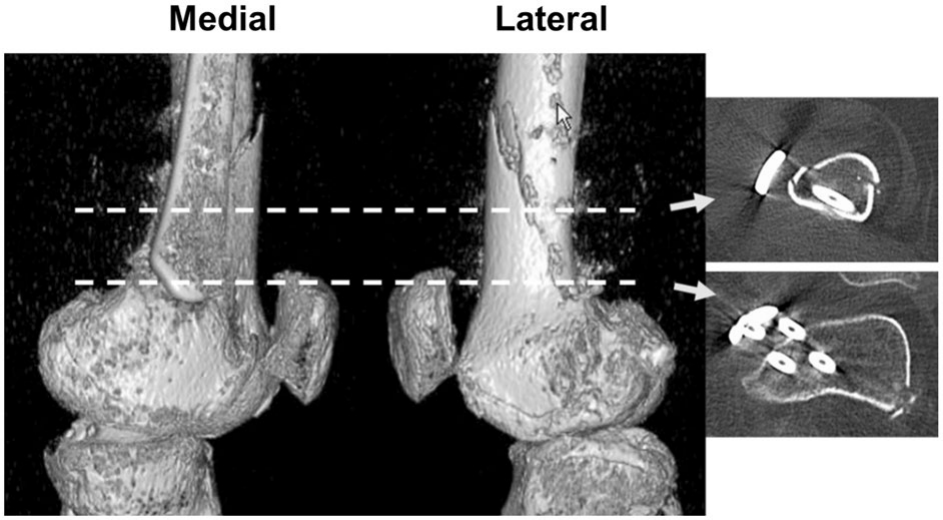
A case of biplanar medial closing wedge osteotomy (MCWDFO). The patient is a 36-year-old female. (Left) Lateral and medial view images of three-dimensional computed tomography, 2 weeks after surgery. The oblique osteotomy line seen on the image from the lateral side corresponds to the osteotomy line of the anterior flange. (Right) Axial images correspond to the level indicated by the broken lines.
